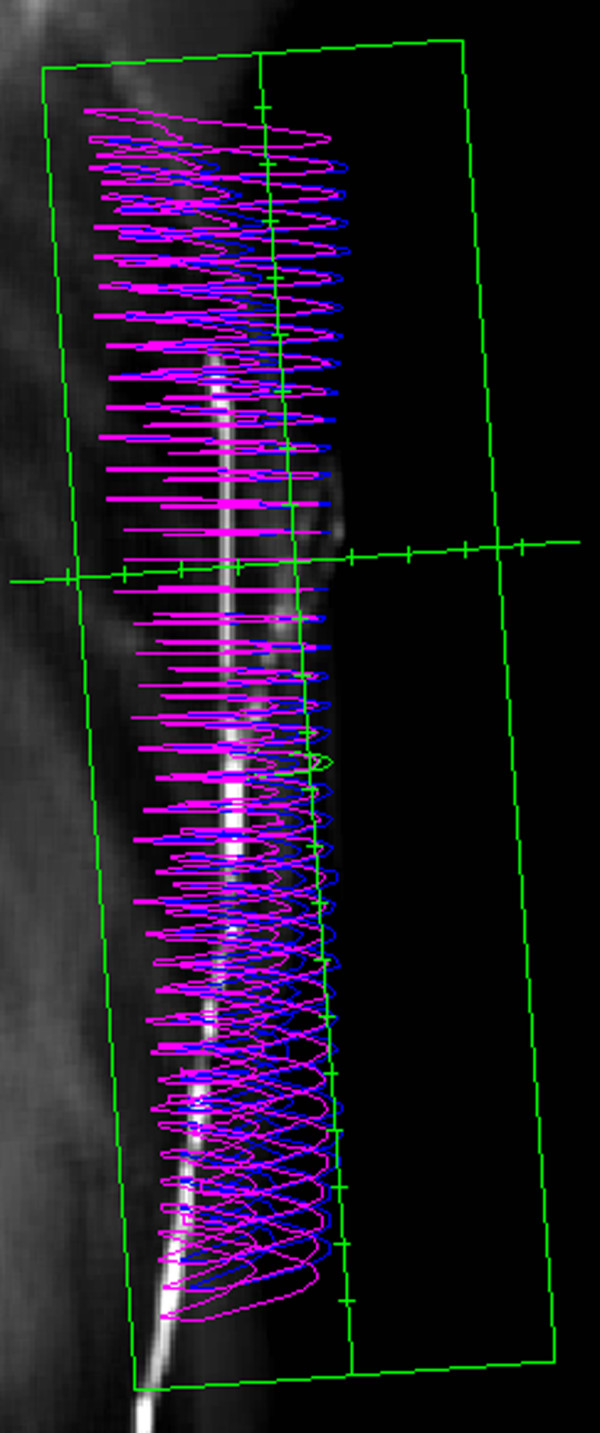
Tangential radiation field on digital reconstructed radiograph.
